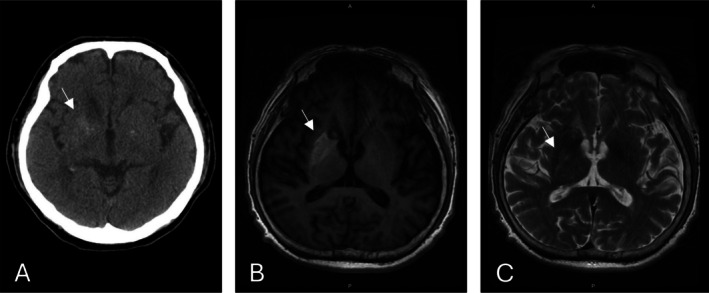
(A) Brain CT without contrast showing hyperdensity in the right lentiform nucleus (white arrow), (B) T1‐weighted fluid‐attenuated inversion recovery MRI of the brain showing a high signal intensity lesion in the right lentiform nucleus (white arrow), (C) T2‐weighted image showing a high intensity signal in the right lentiform nucleus (white arrow).
